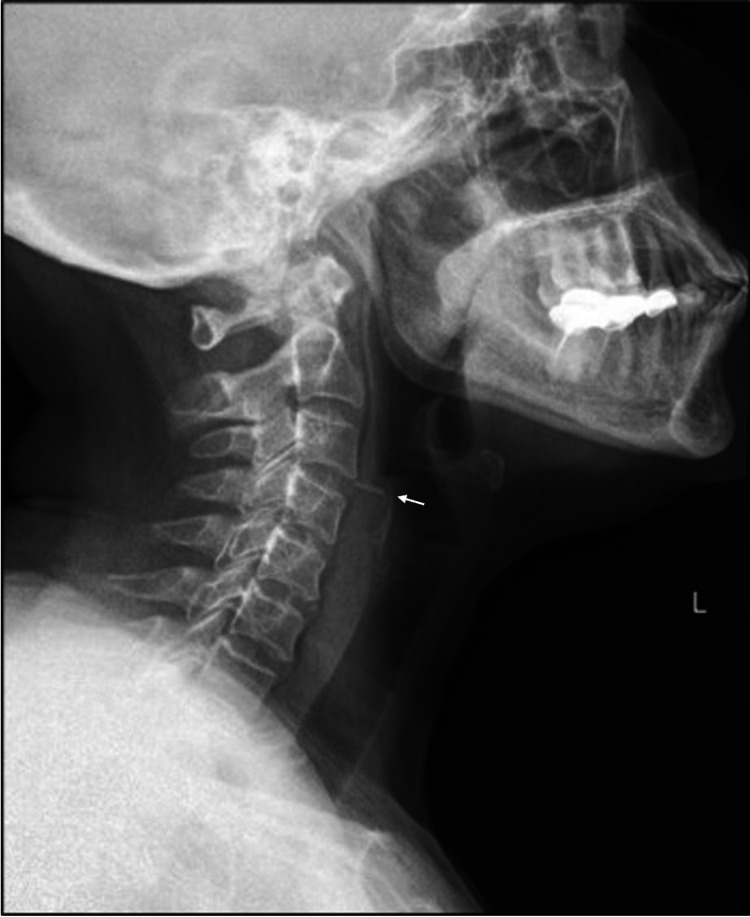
A lateral neck soft tissue x-ray showing a foreign body (arrow) in the hypopharynx with a linear radiolucency in the retropharyngeal space representing free air
